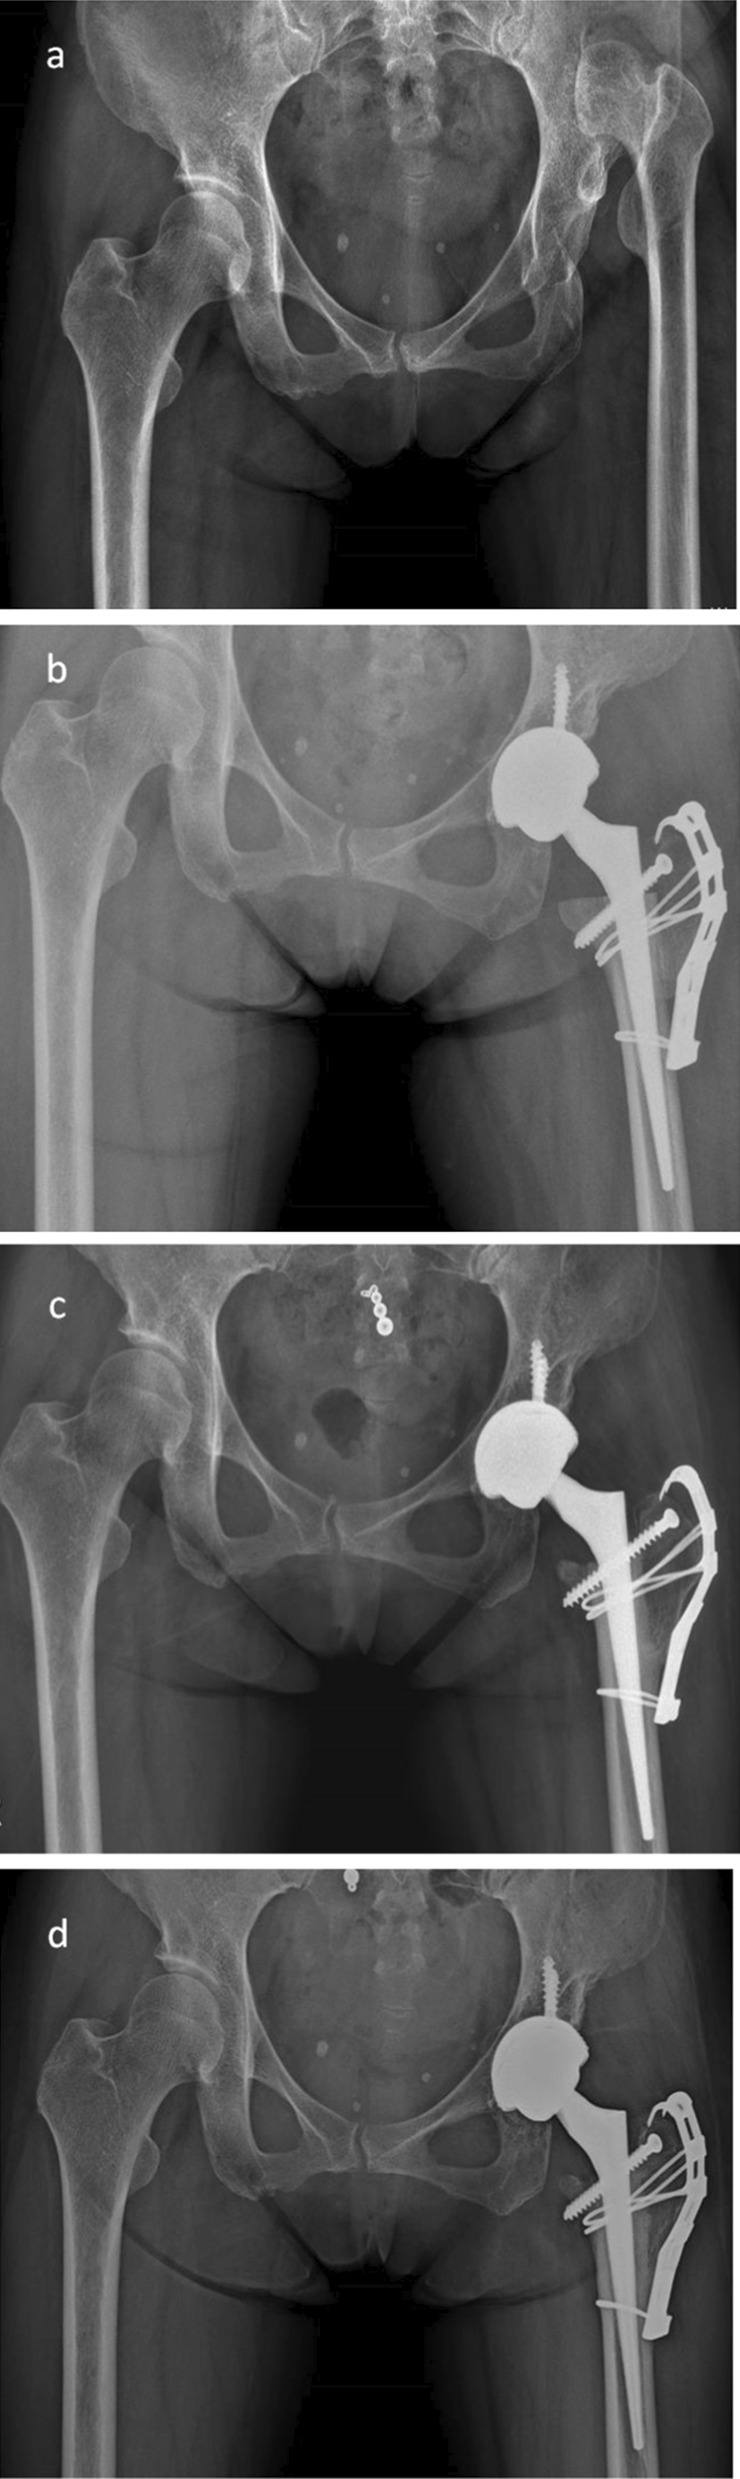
a Preoperative radiograph of a 36-year-old woman with Crowe type IV developmental dysplasia of the left-sided hip. b Bone union at the osteotomy site at 3 months postoperatively; no stem subsidence occurred. c Postoperative 1 year. d Postoperative 6 years
